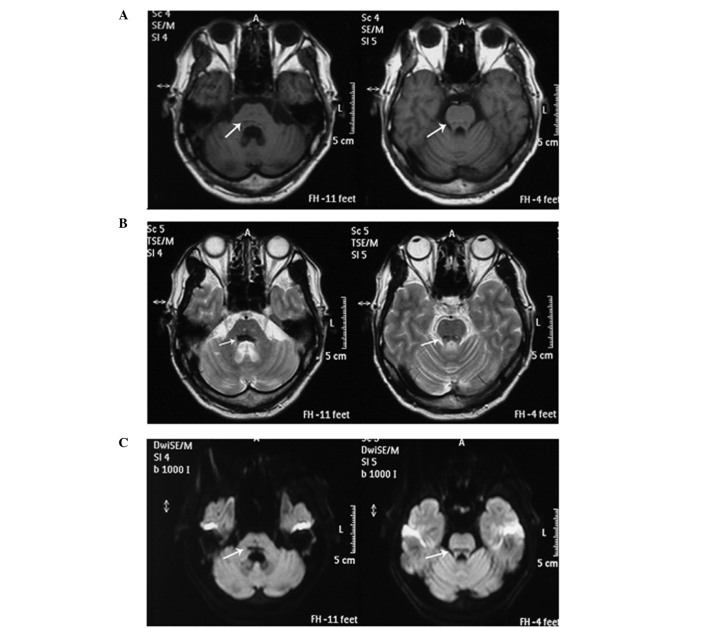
Cranial magnetic resonance images showing areas of linear hypointensity (arrows) on the surface of the pons, using (A) T1-weighted imaging (T1WI), (B) T2-weighted imaging (T2WI) and (C) diffusion-weighted imaging (DWI). Images revealed widened and deepened sulci and gyri, an enlarged ambient cistern and mild cerebellar atrophy. The size of hypointensity due to hemosiderin was larger in the T2WI when compared with the T1WI and DWI.
